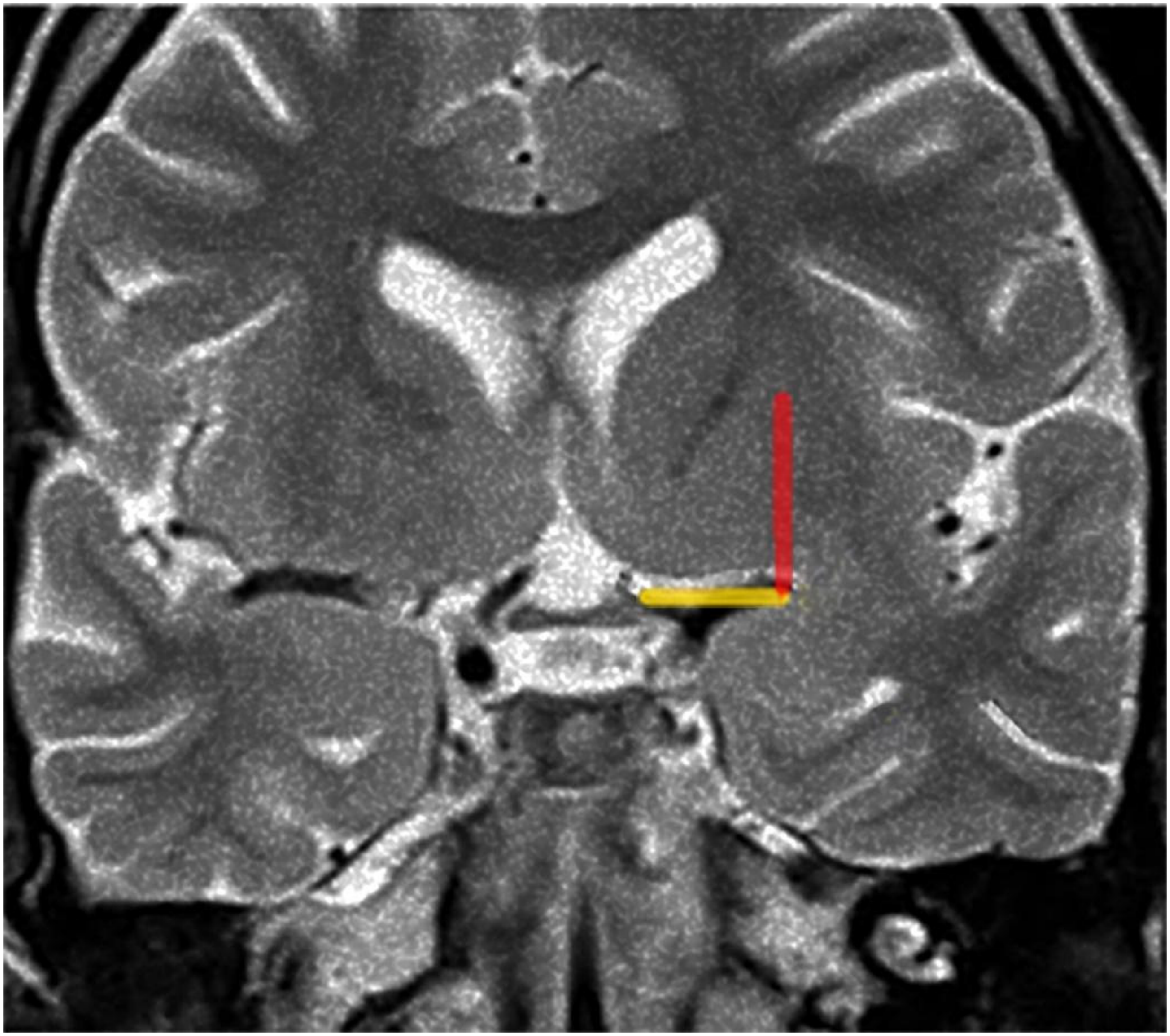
Porto alegre line (red) on coronal T2-weighted MRI reflects indirectaly the lateral limit of the anterior perforated substance, the OC-IR line (yellow). Figure from Isolan et al. (36).
